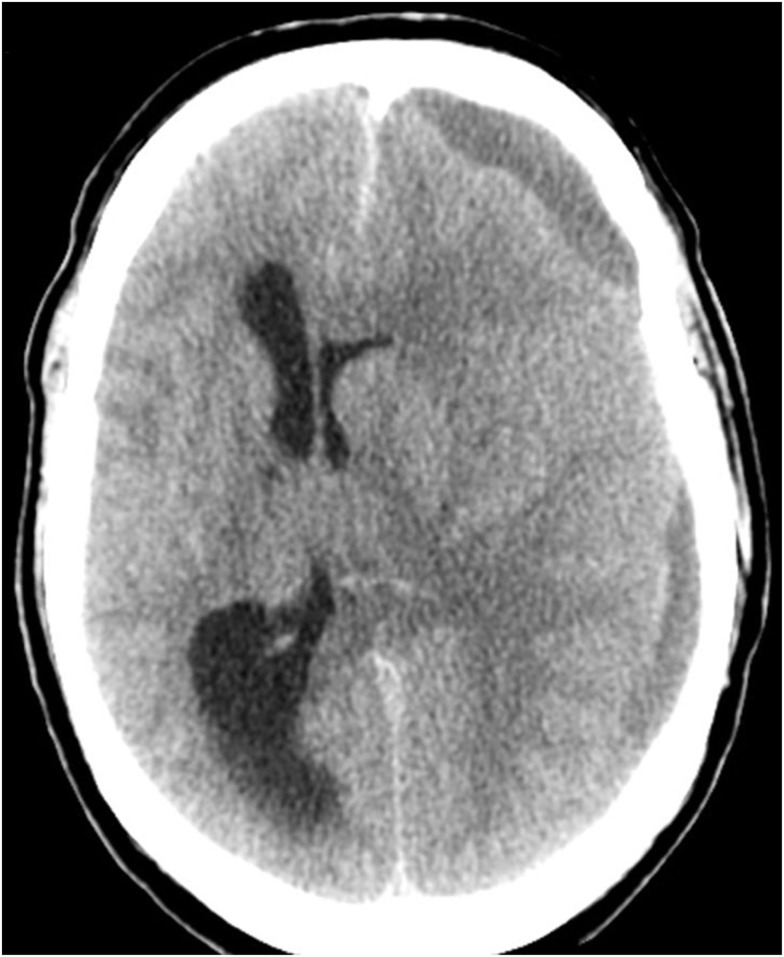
Computerized tomography of the head (CT) on admission showing a left-sided sudural mass and a gross midline-shift of 15 mm.
